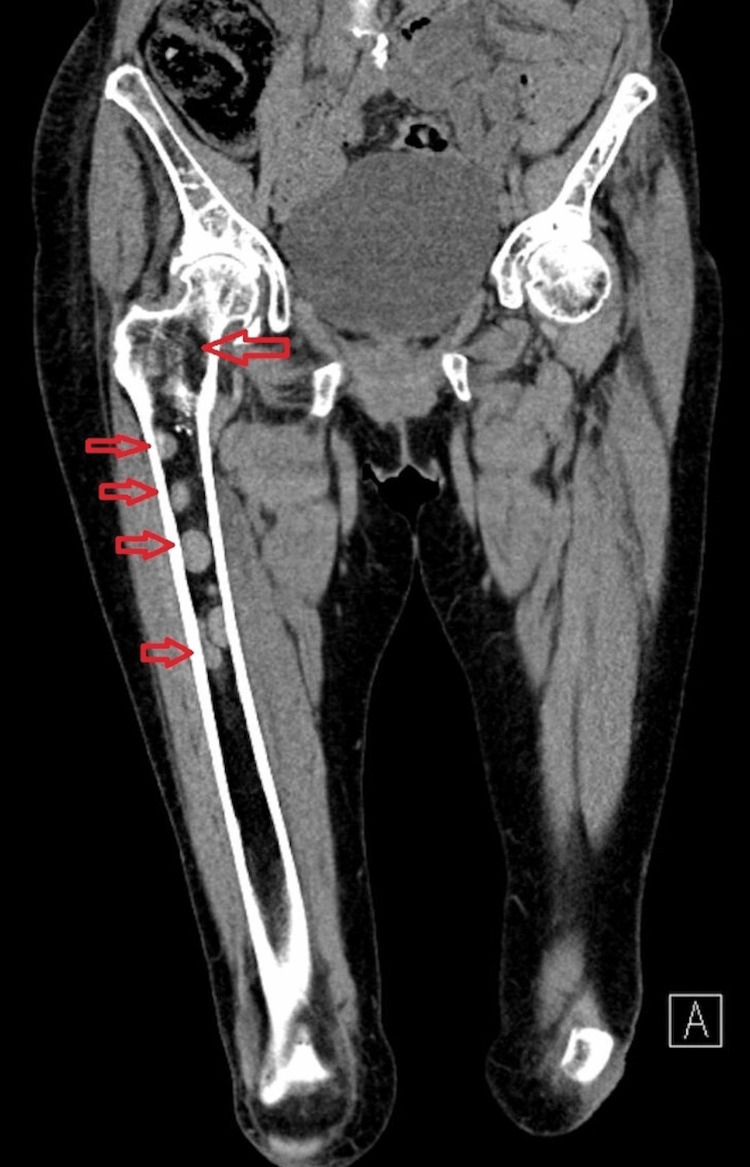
CT scan coronal plane image showing right hip and femur with several multiple myeloma lesions
